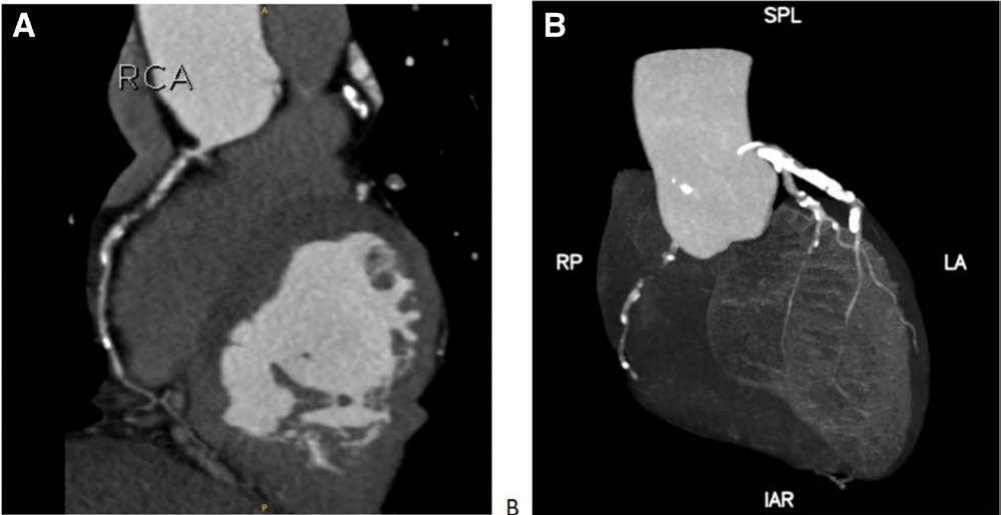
Findings on preoperative coronary computed tomography angiography. IAR = inferior anterior right, LA = left anterior, RCA = right coronary artery, RP = right posterior, SPL = superior posterior lateral.
